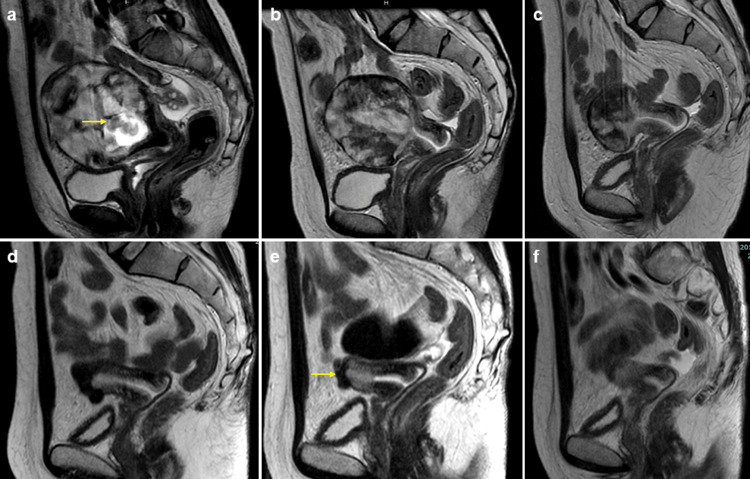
Sagittal T2-weighted contrast-enhanced magnetic resonance imaging (MRI) An indistinct border between the myometrium and placenta is shown, continuing to suggest placenta percreta, although the fetal (yellow arrow) and high-echoic placental components show signs of shrinkage (a). Further reduction in the fetal and placental components is confirmed, and the low-echoic normal myometrium is gradually becoming more defined (b). A follow-up MRI showed no abnormal blood flow in the area, and the low-echo normal myometrium became more distinct (c). The high echoic placental component has been completely replaced by low echoic myometrium, and the uterus has returned to its normal size (d). T2-weighted sagittal contrast-enhanced MRI shows an area of poor contrast at the uterine fundus and the thinning part of the myometrium, which is consistent with the site of the placental villi perforation into the myometrium (yellow arrow) (e). T2-weighted sagittal contrast-enhanced MRI shows that the myometrium has gradually recovered blood flow, and the muscle layer is maintained throughout the entire circumferential uterus (f).
